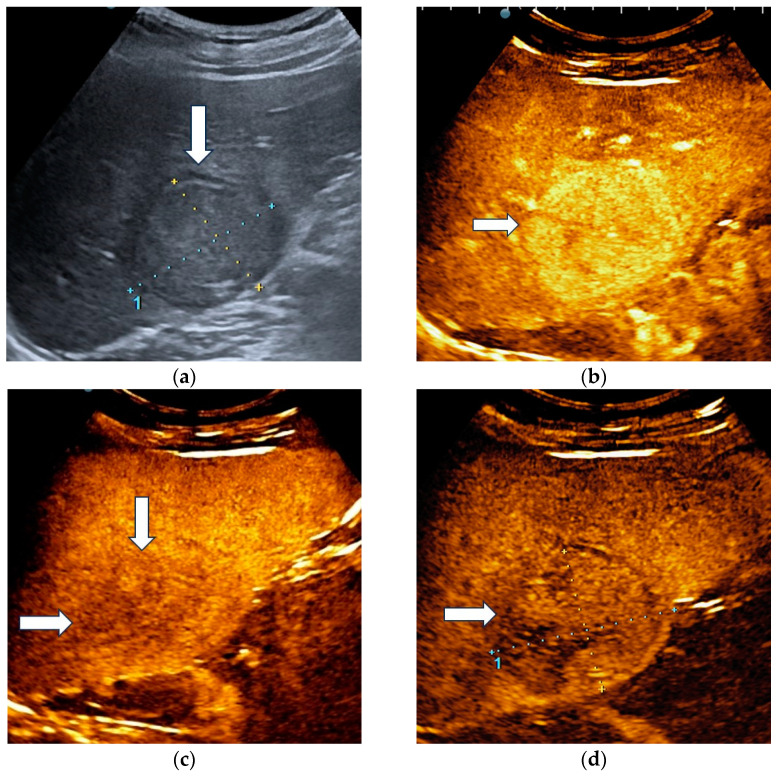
A case of hepatocellular carcinoma with typical CEUS enhancement pattern correctly classified by both clinicians and the AI system. B-mode ultrasound showed an isoechoic lesion (arrow), with a peripheral halo, sized 55/44 mm, located in the right hepatic lobe, segment VI (a). In the arterial phase of CEUS, the lesion showed diffuse hyperenhancement (arrow) (b), followed by washout in the late phase (arrows) with onset later than 2 min (c). At four minutes into the late phase of CEUS (arrow), the washout was still mild (d).
